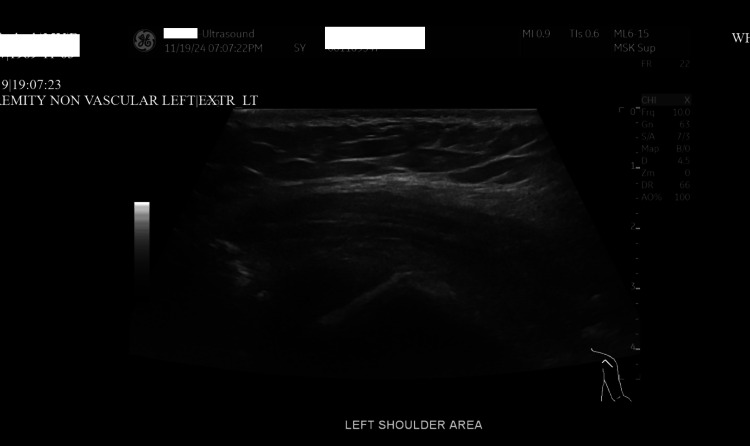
Non-vascular ultrasound of the left upper extremity shows no evidence of soft tissue collection or other abnormalities.
